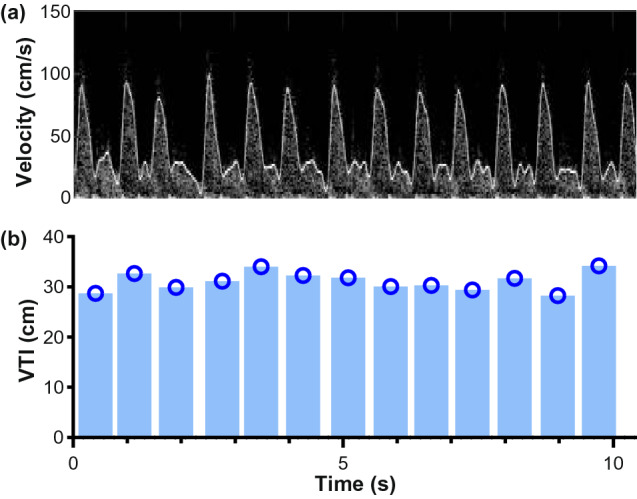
(a) Continuous carotid Doppler waveform monitoring with automatically traced maximum velocity envelope (white line). (b) Beat-to-beat quantification of carotid velocity time integral (VTI).
